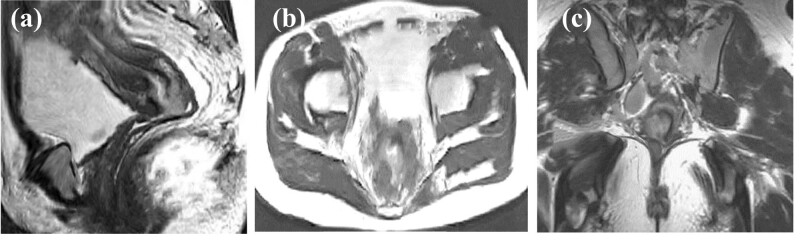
MRI images. (a) Parametrial mass infiltration; (b) enhanced MRI image of the cervix; and (c) parametrial MRI image suggesting high signals on T2WI.
